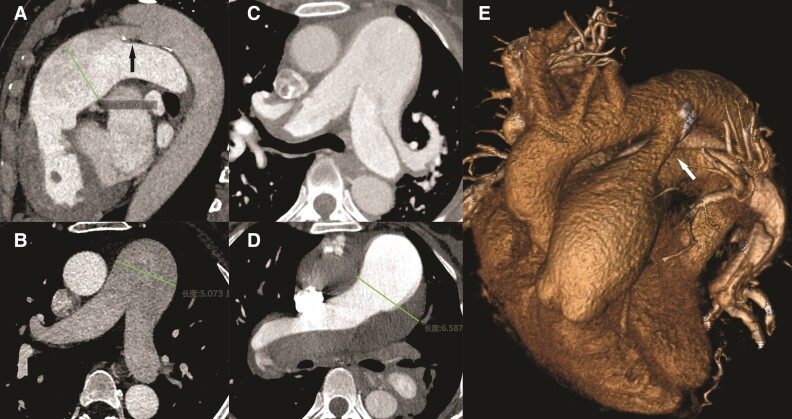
A 52-year-old female was referred to our hospital due to severe chest pain, dyspnoea, and cyanosis. Four years before, she was found to have a patent ductus arteriosus (PDA; arrow, *Panel A*) on contrast-enhanced computed tomography, accompanied by dilation of the pulmonary trunk (5 cm; *Panel B*). No other cardiovascular diseases were discovered. With medical management, her heart condition remained in NYHA functional Class II, and she had full-time employment. The patient, despite the advice of several cardiologists, refused further surgical correction of PDA. On this admission, blood pressure was 90/55 mmHg, and oxygen saturation was 85%. A continuous diastolic and systolic murmur could be heard in the second left intercostal space.
